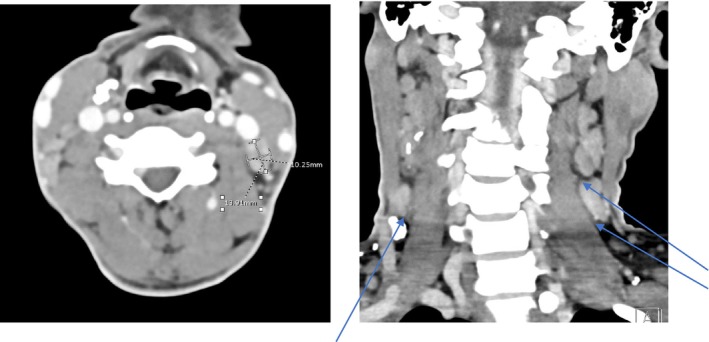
CT neck showing multilevel bilateral enlarged cervical lymph node shown in axial and coronal views.
